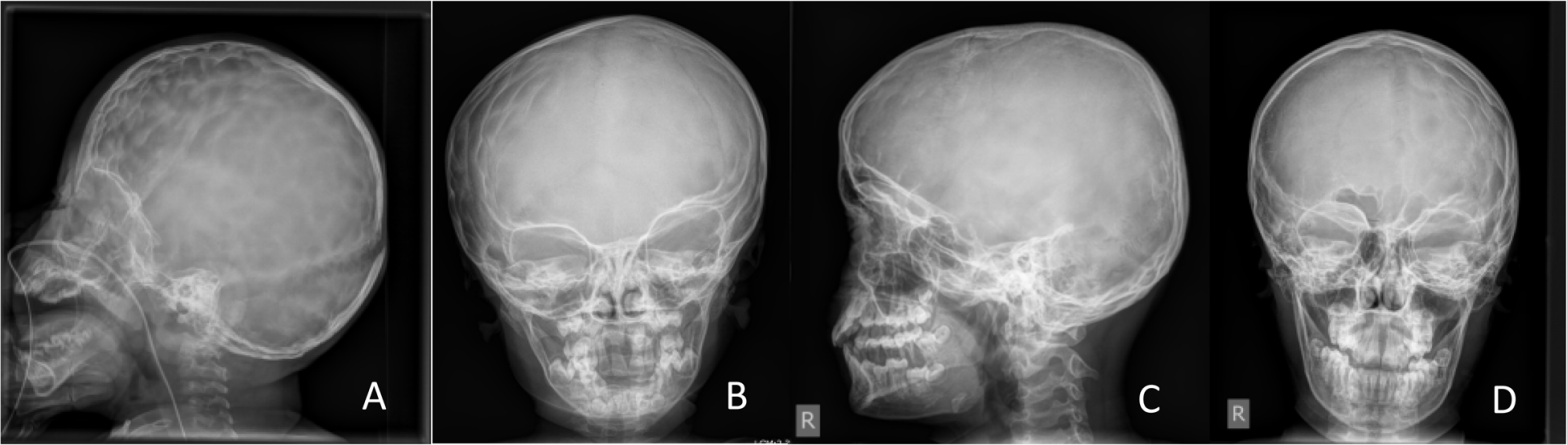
Craniosynostosis in HPP: a: Lateral skull X-ray of a 2 year old girl with premature fusion of both coronal sutures and signs of raised intracranial pressure, copper beaten skull. b: A.p. skull X-ray of a 5 year old girl with premature fusion of the left cornal suture with signs of raised intracranial pressure – impressiones temporal, right side. c and d: lateral and a.p. skull X-ray of a 12 year old boy with premature fusion of the left cornal suture and impressiones digitatae predominant on the left side
